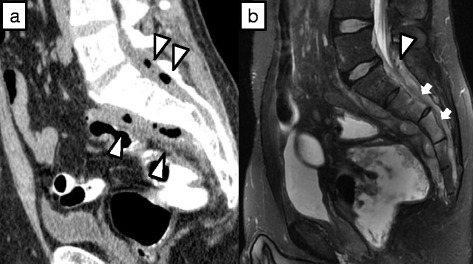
a Sagittal CT shows air density in the spinal canal and soft-tissue density anterior to S1 and S2 (*white arrowheads*). b T2-weighted MR image shows fluid and gas in the epidural space (*arrowhead*), and enhancement of S3 and S4 (*arrows*), indicating inflammatory extension
